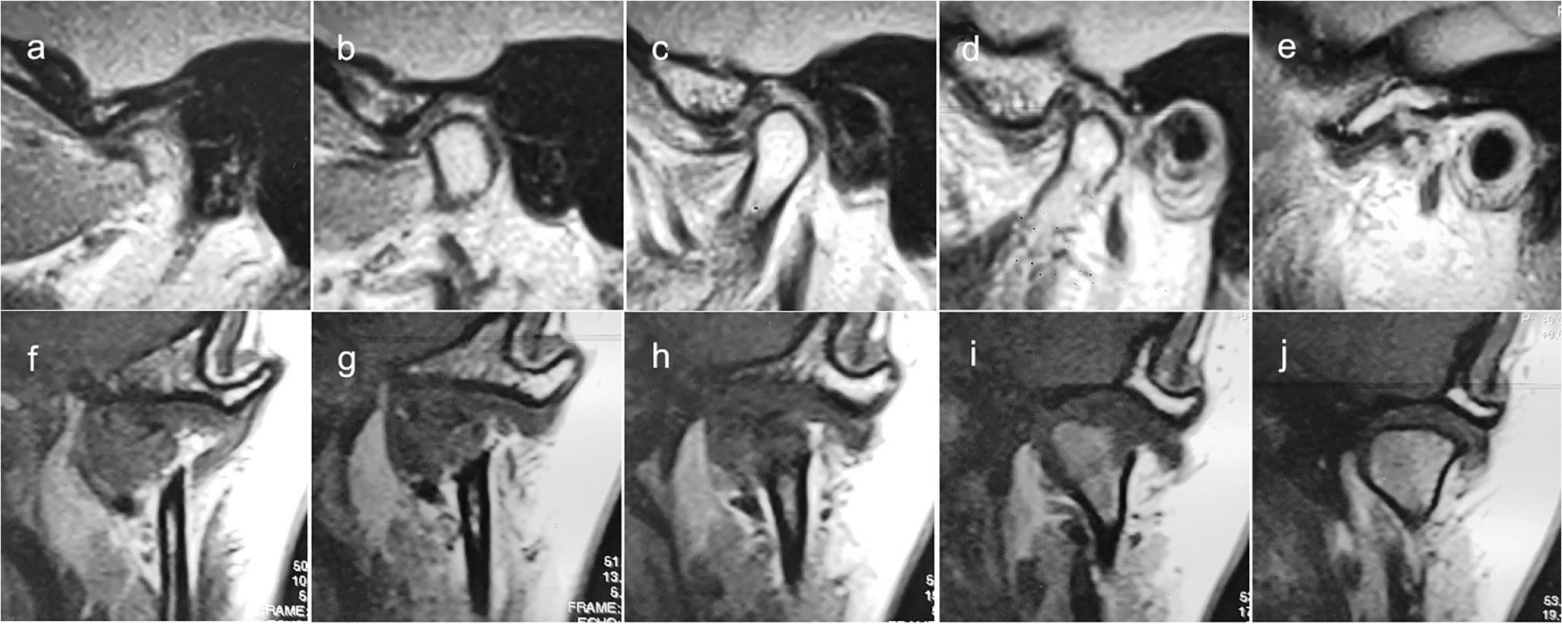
MRI of TMJ with partial anterior disc displacement in the lateral part. a–e Oblique sagittal slices in intercuspal position. f–j Oblique coronal slices in intercuspal position
